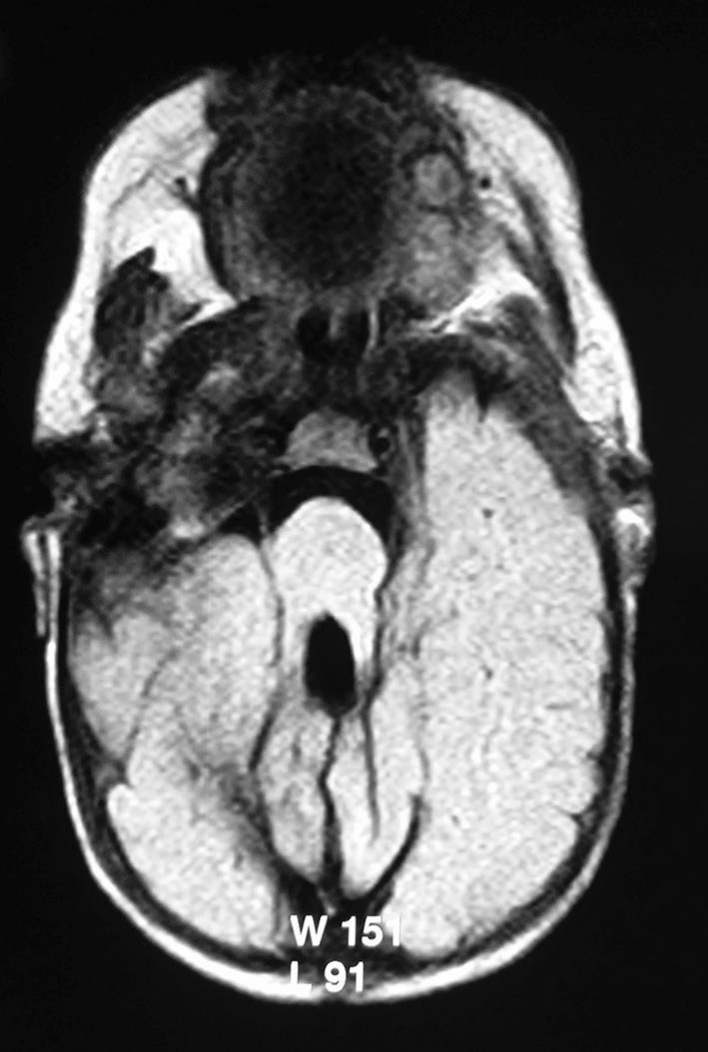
MRI brain, age 56 days. Axial section showing small dysplastic vermis, large rounded superior cerebellar peduncles and absence of decussation of white matter pathways forming the ‘molar tooth’ malformation
